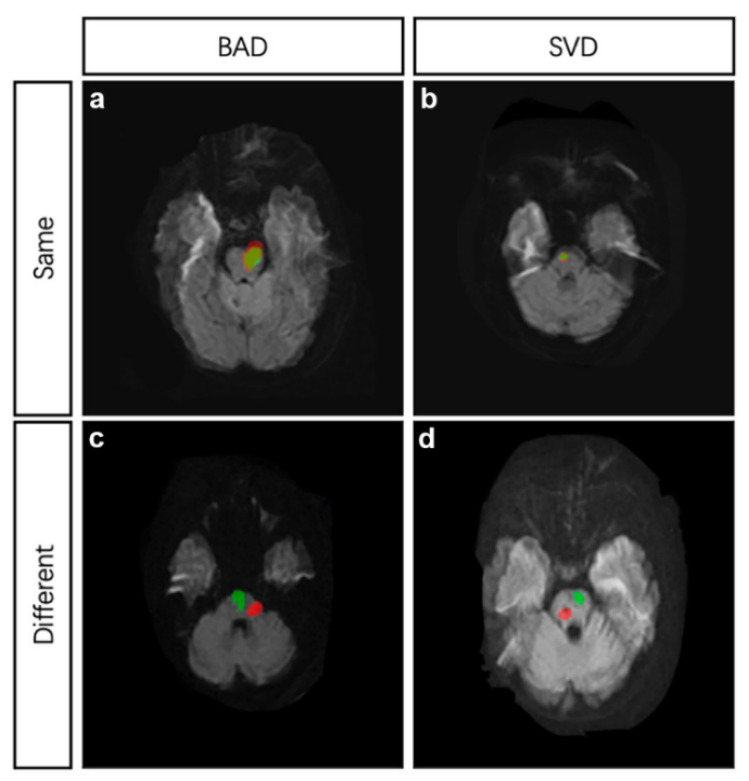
MRI demonstrations of four different conditions. (a), the index stroke and recurrent stroke were both attributed to BAD and located at the same site. (b), the index stroke and recurrent stroke were both attributed to SVD and located at the same site. (c), the index stroke and recurrent stroke were both attributed to BAD but located at different sites. (d), the index stroke and recurrent stroke were both attributed to SVD but located at different sites. Red area indicates the index infarction while green area indicates the recurrent infarction. BAD, branch atheromatous disease, SVD, small vessel disease.
